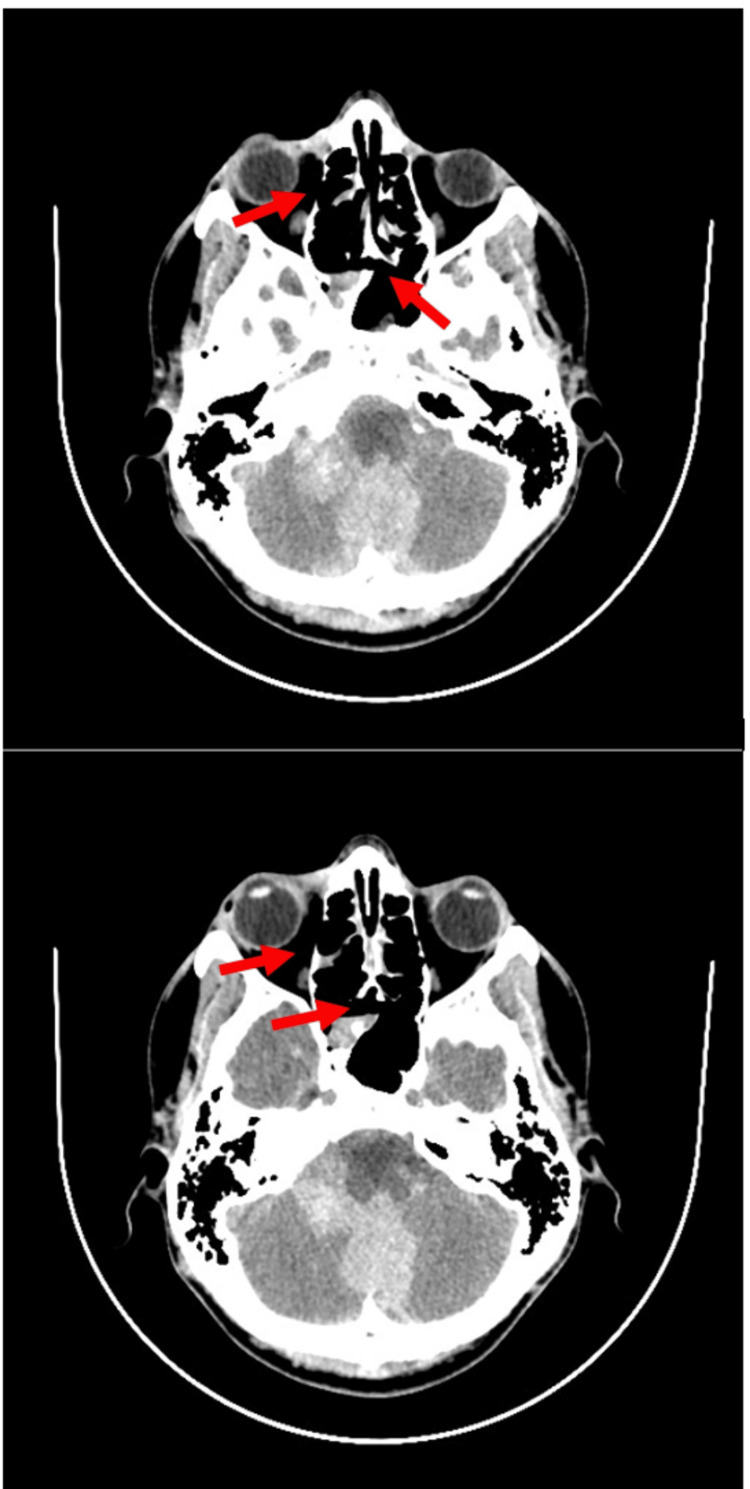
CT scan from the day of admission showing multiple facial fractures (top) and subdural hematoma (bottom) due to scooter accident
